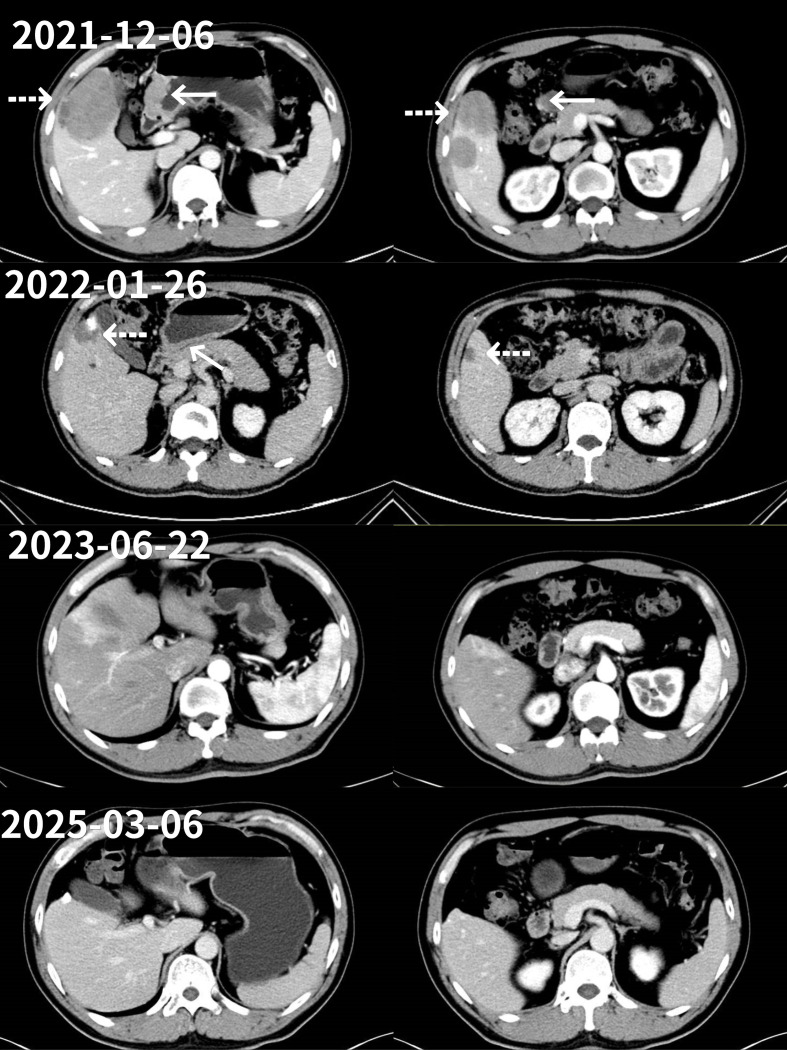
CT images obtained on December 6, 2021, upon the patient’s admission, showing multiple hypodense nodules and masses in the liver with ill-defined borders. The largest mass measures approximately 7.1cm×5.1cm, and shows rim enhancement on contrast-enhanced scans.The remaining images are follow-up CT scans during the treatment period. Comparison shows that the patient’s liver metastatic lesions gradually improved and eventually disappeared completely.
